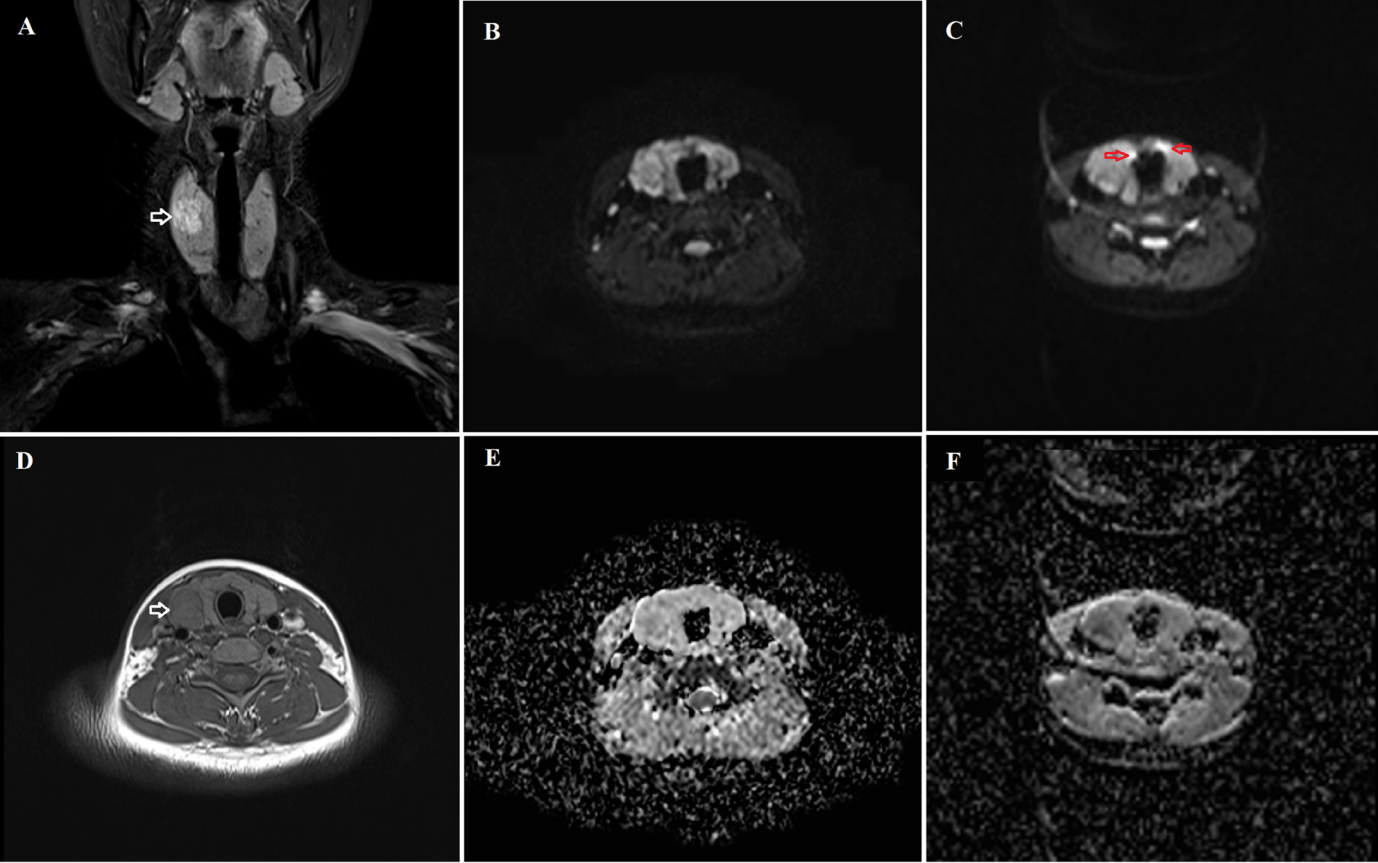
Comparison of the iShim-EPI and 3D-Shim-EPI sequences in a 26-year-old female with a thyroid nodule in the right thyroid lobe (A) is a T2-weighted image with fat saturation and (D) is a T1-weighted image. Hyperintensity and hypointensity characteristics of the thyroid nodule were observed on these images (white arrows). The iShim-EPI DWI image had b = 500 s/mm^2^ (B) and ADC map (E). 3D-Shim-EPI DWI image with b = 500 s/mm^2^ (C) and ADC map (F). Susceptibility (red arrows) and ghost artifacts are shown on the 3D-Shim-EPI images, whereas improved image quality was observed using the iShim-EPI technique.
